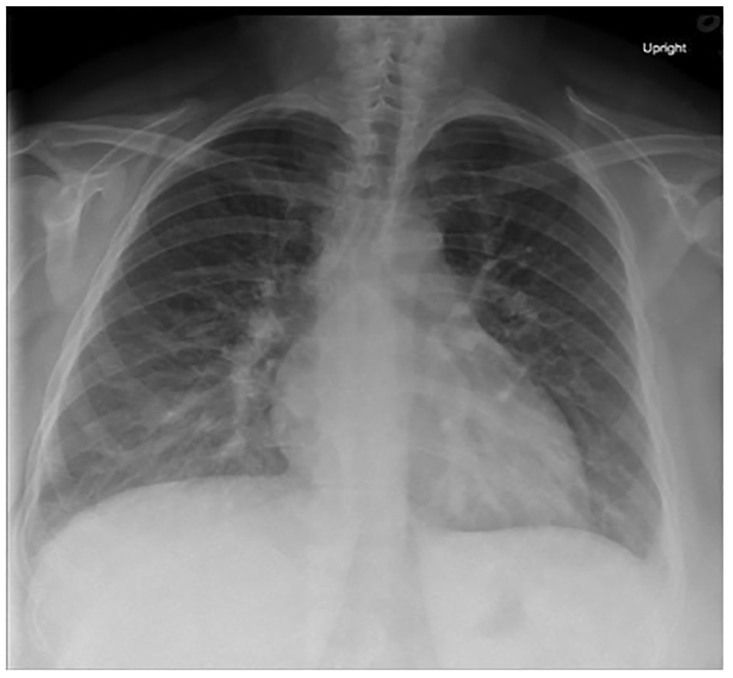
Initial anterior-posterior chest radiograph taken shortly after arrival to the emergency department.
